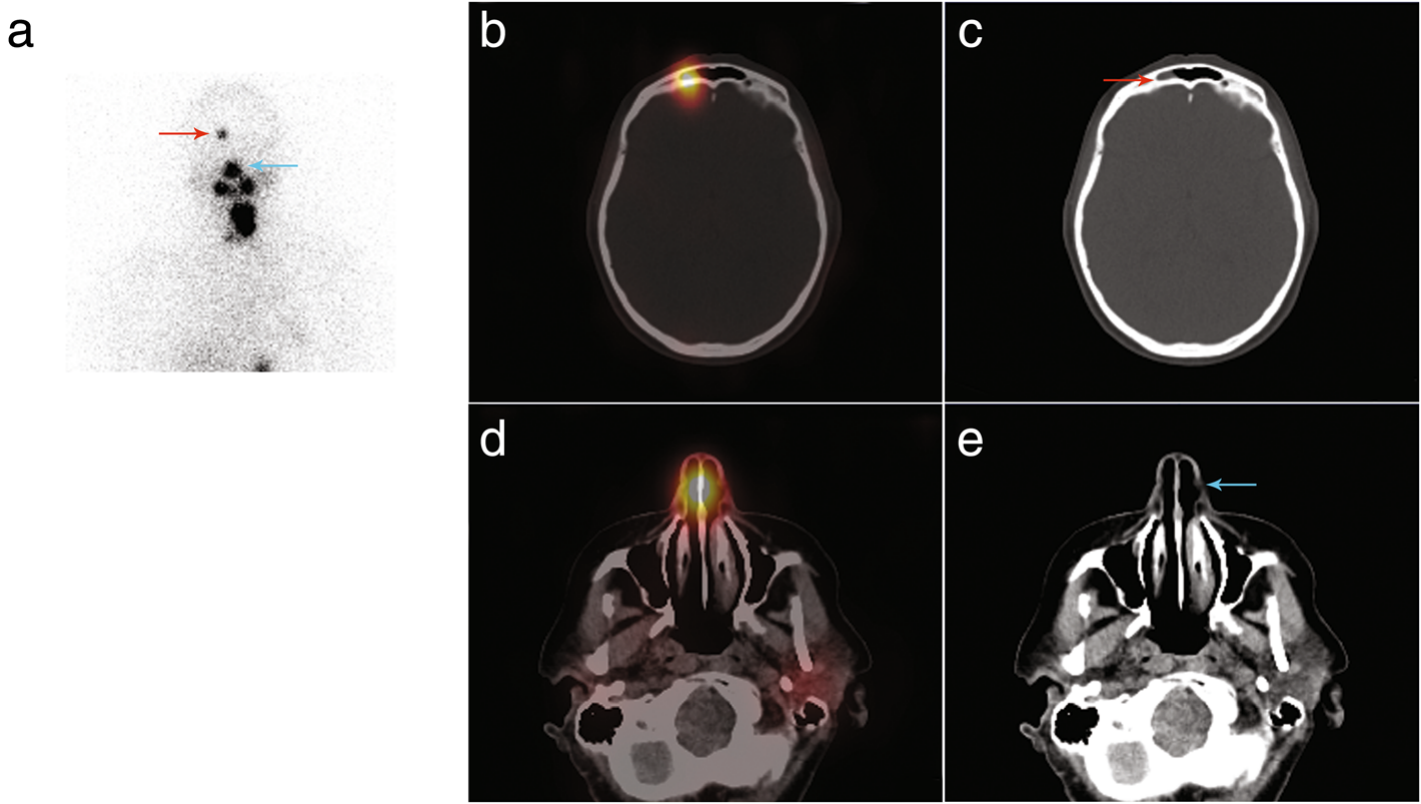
Planar acquisition (anterior view, Panel a), fused (Panel b) and CT (Panel c) images of SPECT/CT acquisition displaying RAI uptake in the right part of the frontal sinus with mucosal thickening on CT scan slices (*red arrow*). RAI uptake is also visible in the nose (Panels a, d and e, *blue arrow*)
